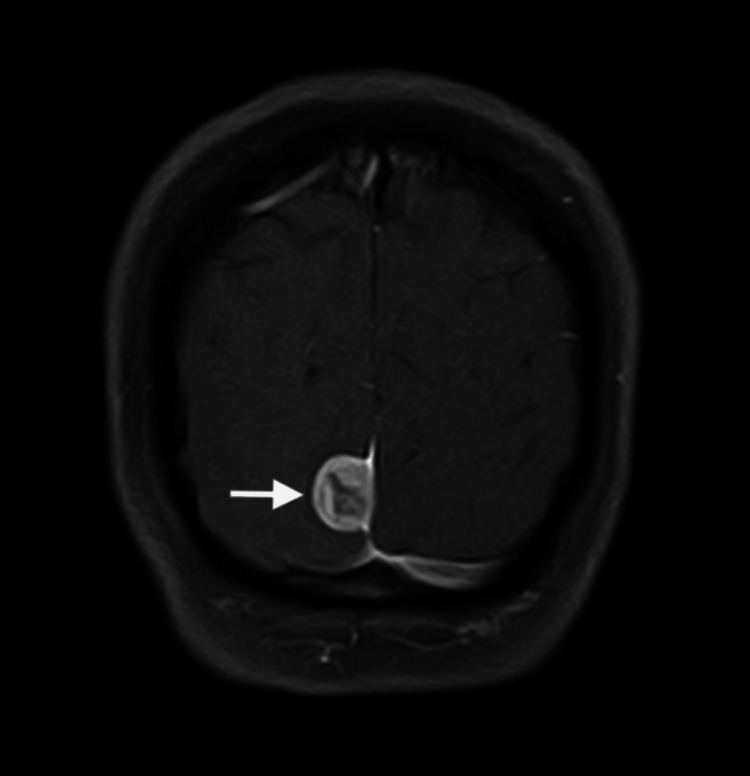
Preoperative magnetic resonance imaging scan at the T1 coronal view level. Arrow is pointing toward the lesion.
